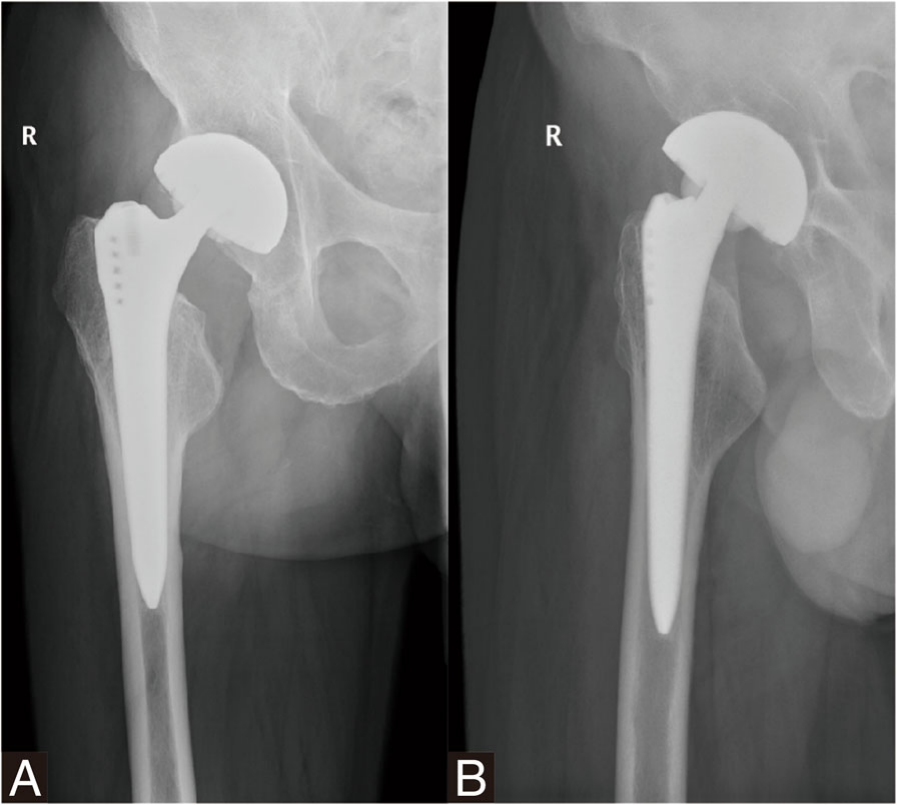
Radiograph showing fracture of the ceramic femoral head with marked fragmentation (a) Anteroposterior view. b Lateral view
